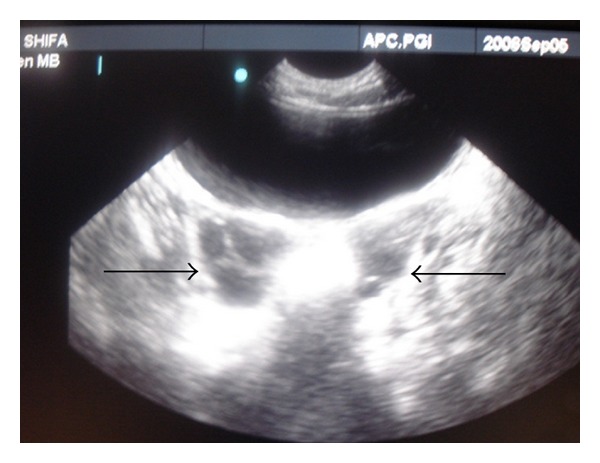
Ultrasonogram showing multicystic ovaries (Rt > Lt).
